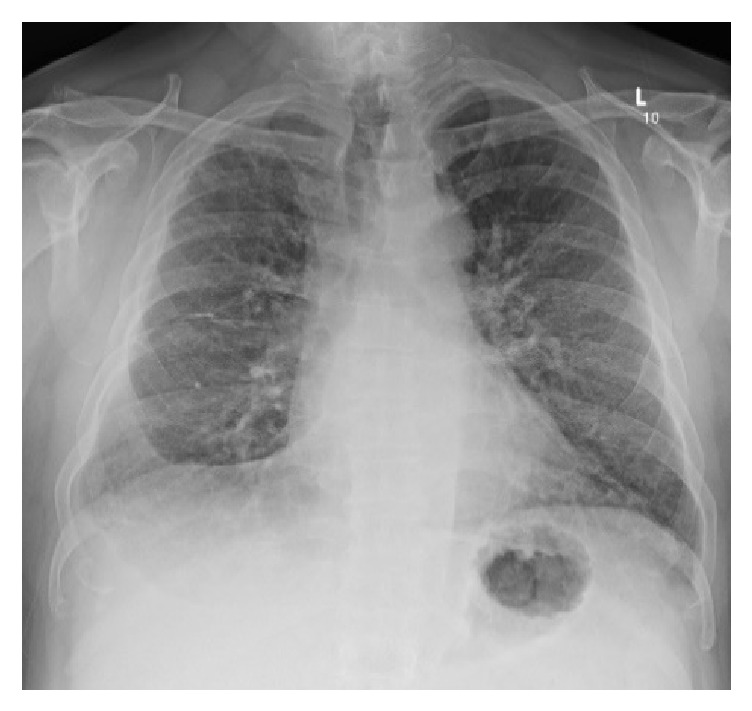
Anteroposterior chest X-ray 10 days after acute exacerbation and treatment with glucocorticoids. Right pleural effusion resolved with improvement in interstitial opacities.
